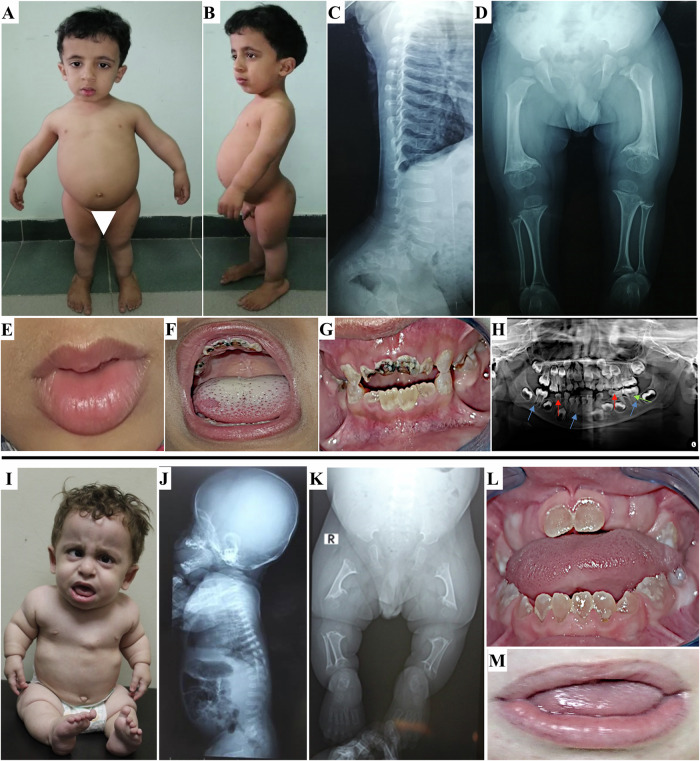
Patient 1 at the age of 5 years. A, B Frontal and lateral views showing relative macrocephaly, frontal and parietal bossing, high forehead, flat philtrum, low set ears, short neck, short narrow chest, depressed lower sternum, distended abdomen, exaggerated lumbar lordosis, broad joints, genu valgum and flat feet. C, D X-ray spine lateral view and lower limbs (AP view) showing mild platyspondyly, globular shaped vertebrae, flat acetabulum, the greater trochanter of the left femur was separated and posteriorly displaced, rounded femoral head, broad femoral neck, wide knee space, globular epiphysis around knees, both fibulae were proximally short, broad cupped frayed sclerotic metaphysis and short bowed long bones. E Microstomia and everted fissured lower lip. F Very high arched palate and geographic tongue. G Opalescent dentin and anterior open bite. H Panoramic radiograph showing bulbous crowns of deciduous teeth, needle like roots anterior lower teeth with obliterated pulp (blue arrow), thin roots of molars (blue arrows), wide pulp chamber (red arrow), pulp stone (green arrow), premature eruption of lower right first premolar, and very thin dentin layer. Patient 2 at the age of 1 year and 9 months. I Frontal view showing relative macrocephaly, high forehead, frontal and parietal bossing, squint, bulbous nose, simple ears, low set ears, short neck, short narrow chest, pigeon chest, flared ribs and skin creases. J, K Skeletal survey showing platyspondyly, globular shaped vertebrae, flat acetabulum, absent femoral head, dumbbell shaped short long bones, wide knee spaces, faint around knees epiphyseal ossification, broad cupped frayed metaphysis, and bone rarefaction. L Intraoral photo shows opalescent dentin of the erupted deciduous dentition. M Thin upper lip, fissured lower lip, open mouth and protruded tongue
